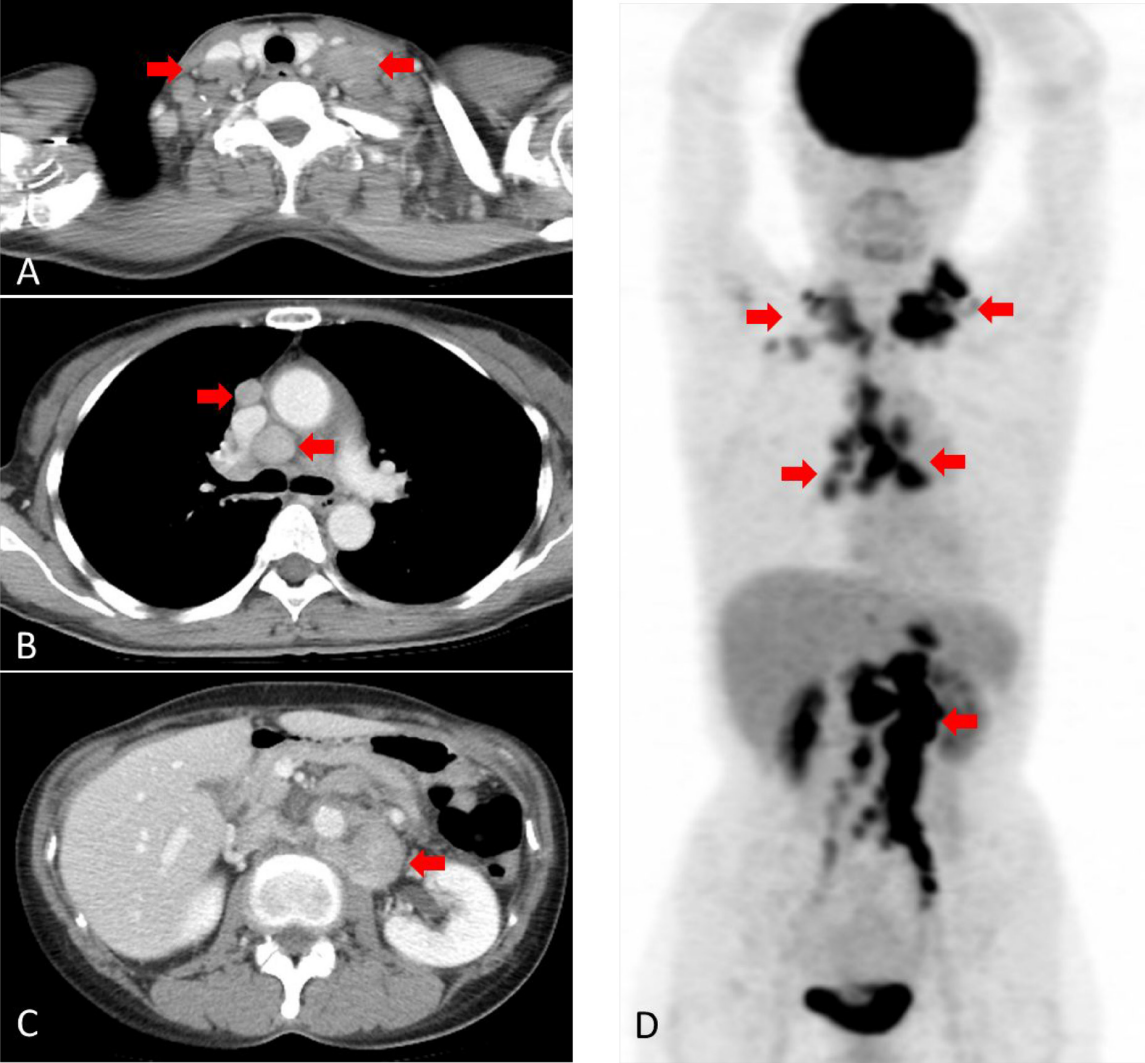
Computed tomography (CT) and [18F]-fluorodeoxyglucose (FDG) positron emission tomography (PET) before treatment (A) CT scan showing swelling of bilateral neck lymph nodes (arrowhead). (B) CT scan showing swelling of the mediastinal lymph nodes (arrowhead). (C) CT scan showing swelling of the abdominal lymph node (arrowhead). (D) FDG-PET scan showing high FDG uptake at the same lymph nodes detected via CT (arrowhead).
